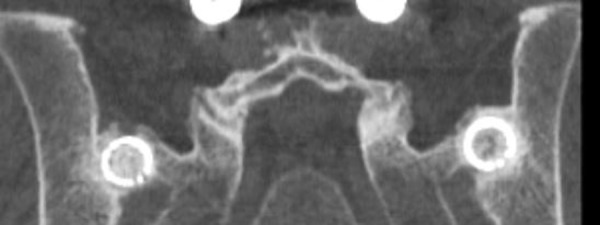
Coronal CT of bilateral posterior lateral oblique fusion
